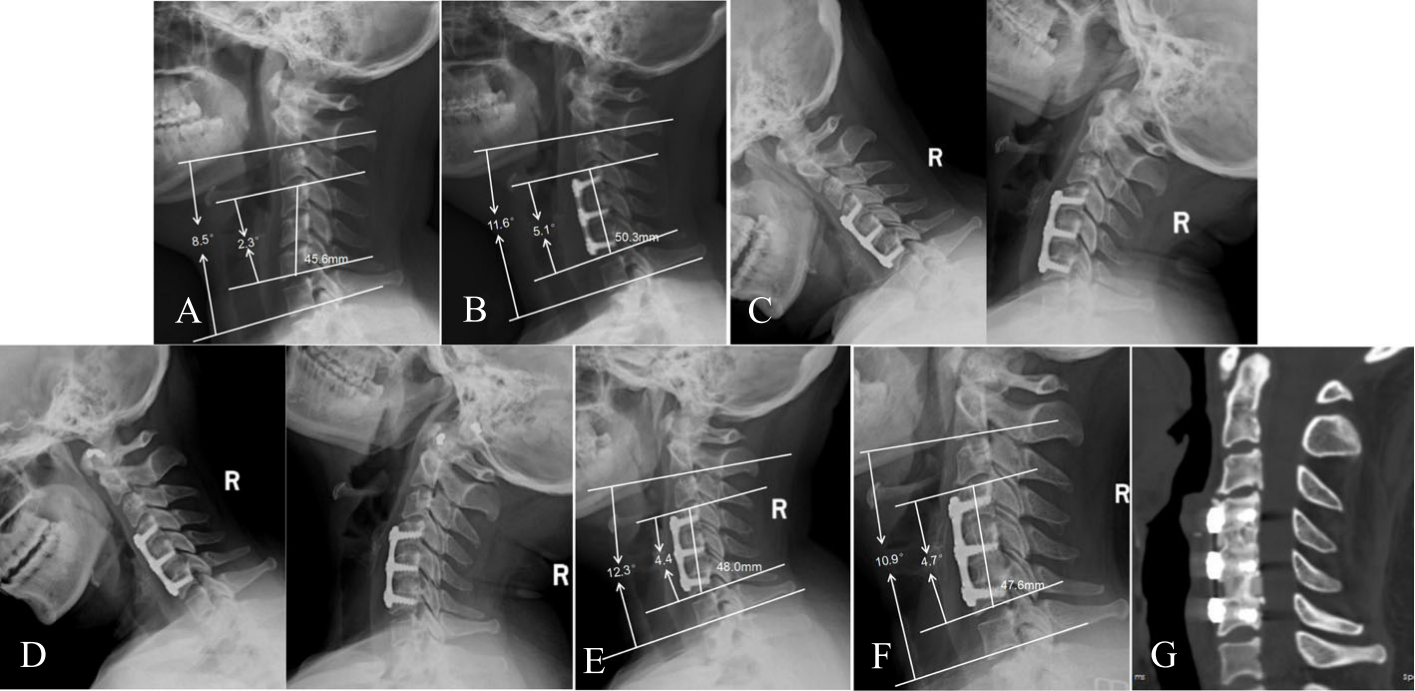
A 50-year-old female patient underwent double-level ACDF due to cervical myelopathy. A mixture of bone dust and morselized bone was used as an n-HA/PA66 cage-filling material in this patient. A Preoperative lateral radiograph of the cervical spine showing narrowing of the C4/5 and C5/6 disc spaces. B Postoperative lateral radiograph showing that the n-HA/PA66 cage increased the FSH, SSA, and C2–7 lordotic angle. C Three-month follow-up flexion-extension lateral radiograph showing the absence of motion between the spinous processes. D Six-month follow-up flexion-extension lateral radiograph showing solid fusion. E, F One-year and final follow-up lateral radiographs showing mild cage subsidence in both segments; the FSH, SSA, and C2–7 lordotic angle remained stable. G Three-dimensional CT at the 36-month follow-up time showing grade 5 fusion in both segments
